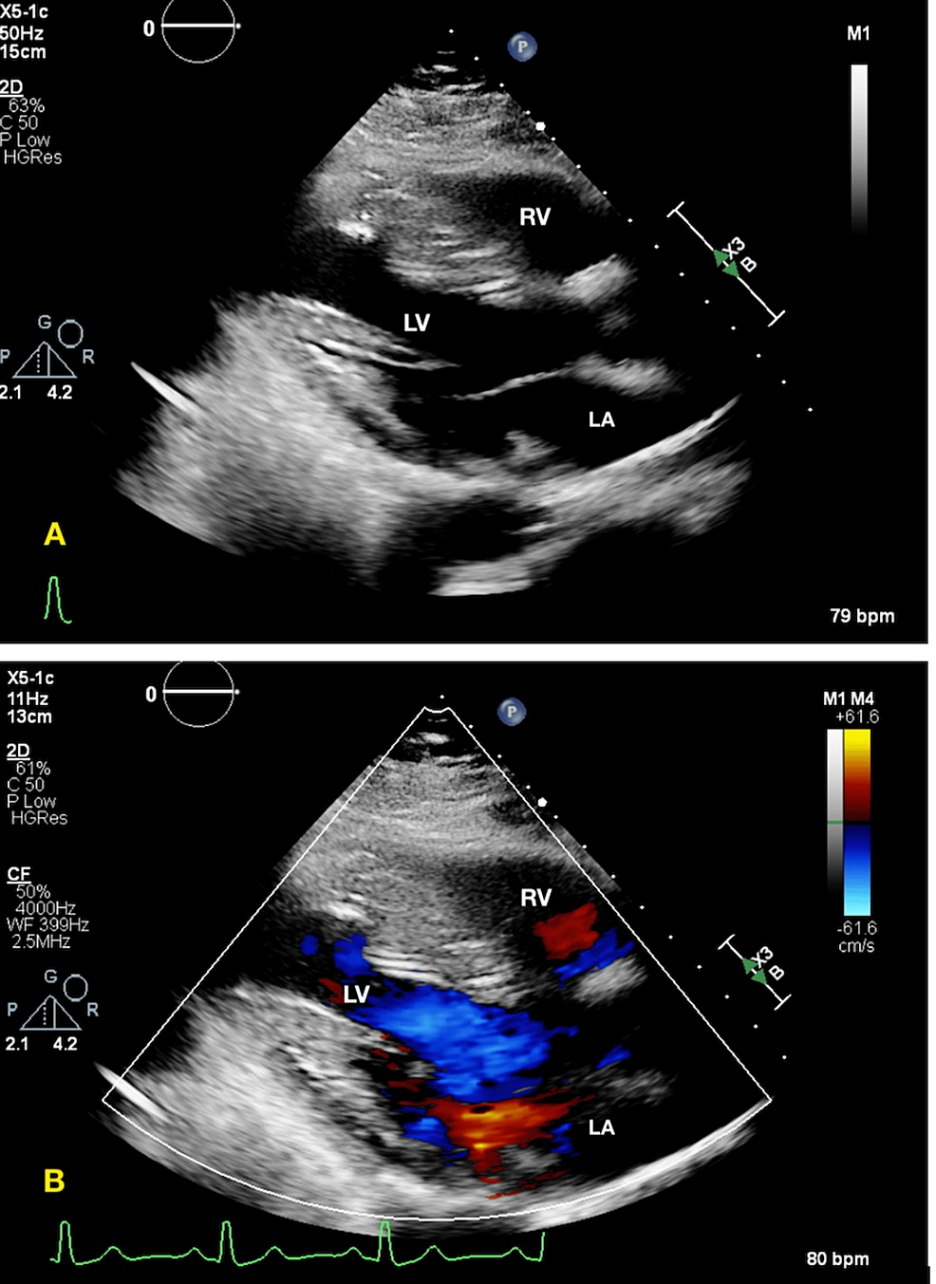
2D Transthoracic Echocardiogram in Parasternal Long Axis demonstrating normal systolic and diastolic function. A: Without doppler flow. B: With doppler flow RV: right ventricle, LA: left atrium, LV: left ventricle
